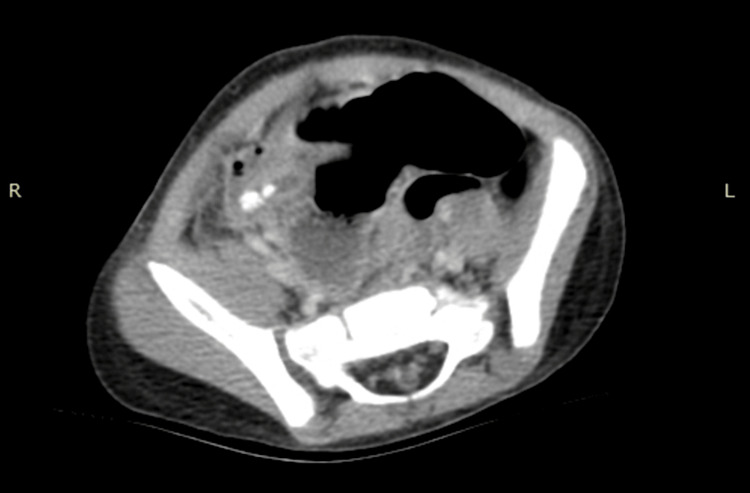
CT abdomen and pelvis demonstrating a dilated appendix with surrounding inflammation and trace fluid.
